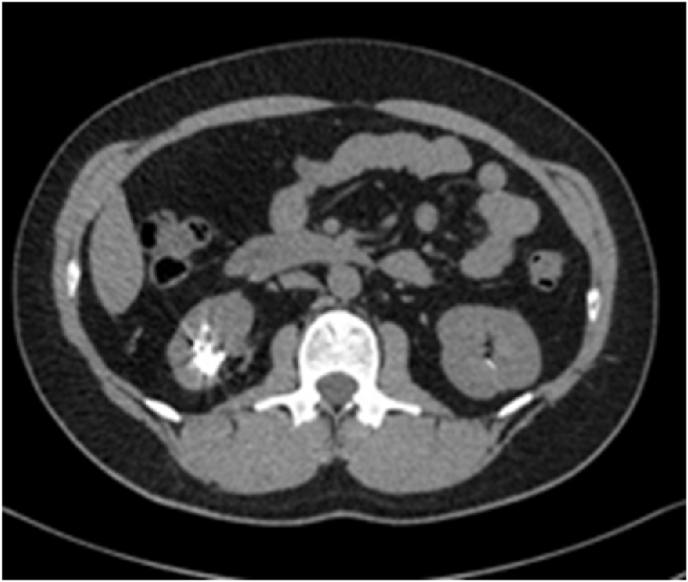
CT abdomen/pelvis (axial view) demonstrated a right renal calculus with an adjacent hyperdense linear density.
